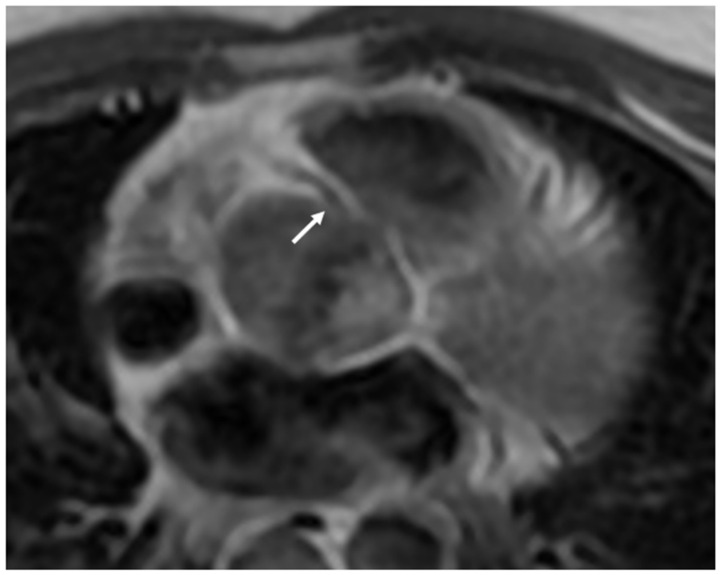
Right coronary artery origin from left sinus with intraarterial course (arrow) in magnetic resonance imaging.
